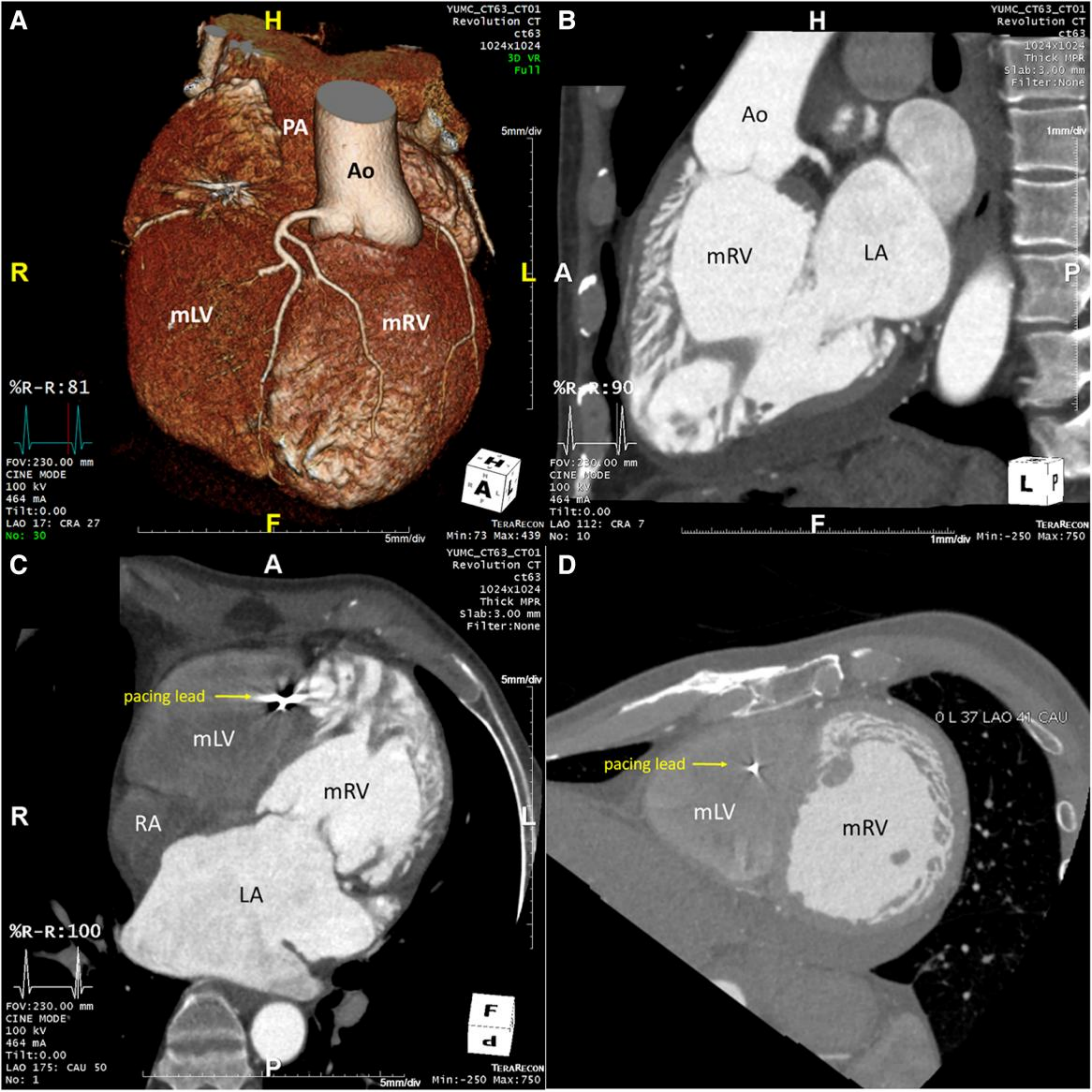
Cardiac CT of a 57-year old male patient confirming the diagnosis of ccTGA. *(A)*: 3D-anatomical reconstruction revealing L-transposition of the great arteries with the aorta in left anterior position arising from the systemic morphologic right ventricle. *(B)*: CT 3-chamber view revealing progressive RA- and RV-dilation with a sRV in sub-aortic position and a tricuspid atrioventricular valve. *(C)*: CT 4-chamber view revealing RA- and RV-dilation as well as the pacemaker-wire in the apical subpulmonary LV. *(D)*: CT short axis view at the papillary muscle level revealing the left sided and dilated systemic RV in leftward position. Ao, Aorta; LA, left atrium; mLV, morphologic left ventricle; mRV, morphologic right ventricle; PA, pulmonary artery; RA, right atrium.
